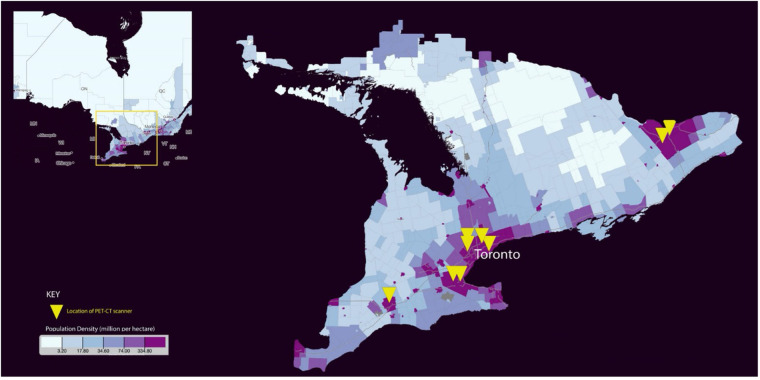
Population density distribution and location of PET/CT scanners across Ontario [*Map created from Statistics Canada* (30)].
